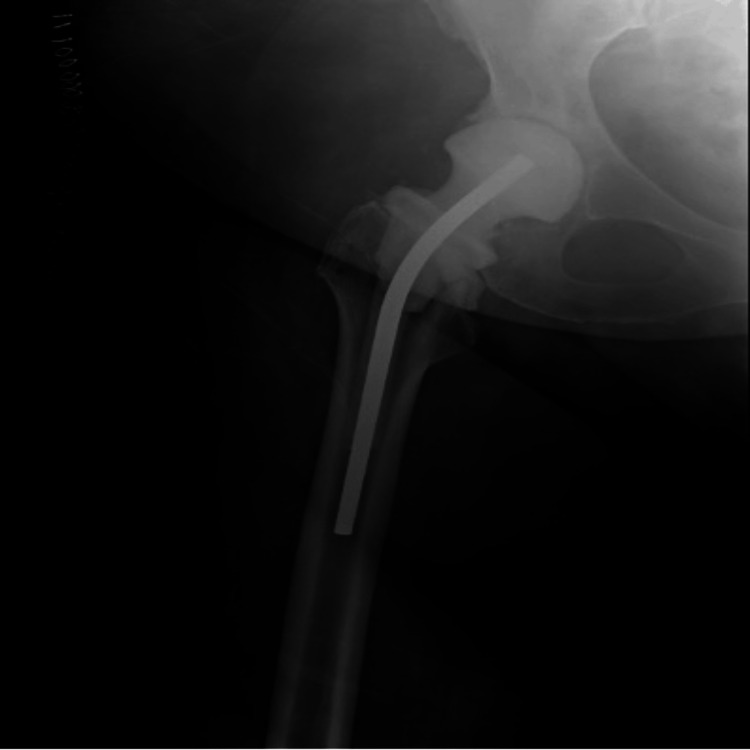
Plain radiograph after failed DAIR procedure DAIR: debridement, antibiotics, and implant retention
